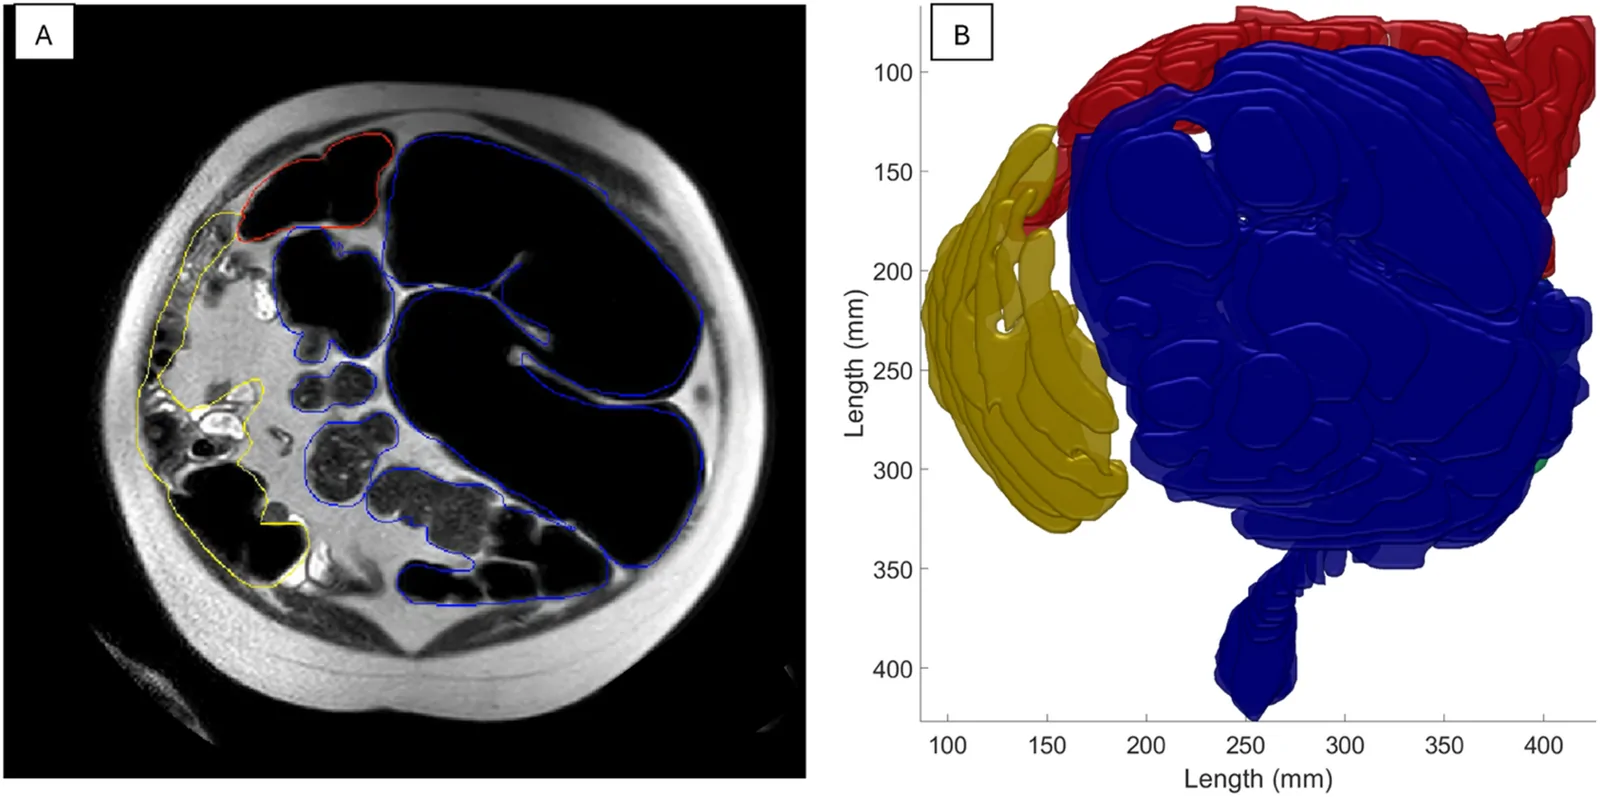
A Coronal T2-weighted MRI of the abdomen showing an abnormally large colon in a patient with spinal cord injury. The colon volume is segmented into the colon segments; the ascending colon is yellow, the transverse colon is red, the descending colon (not shown), and the sigmoid colon/rectum in blue. B 3D-model of the segmented colon volume with the same color codes as shown on the MRI
